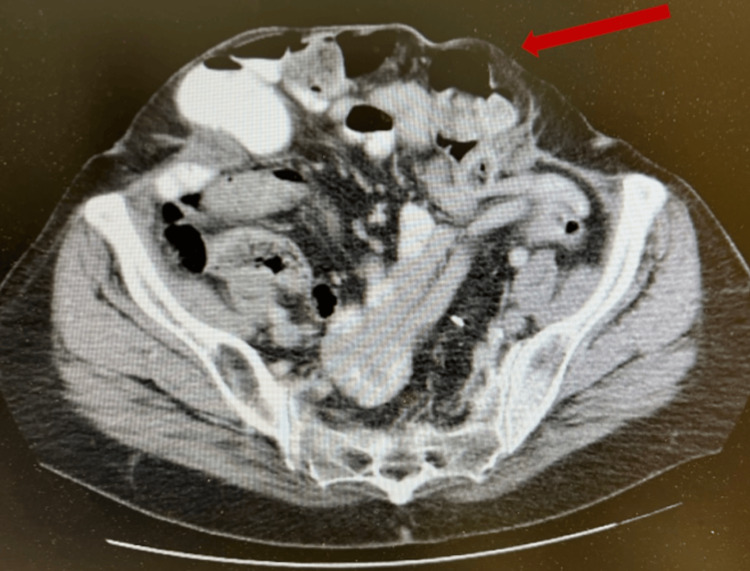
CT scan demonstrating a giant ventral hernia with significant loss of domain, characterized by protrusion of abdominal contents beyond the confines of the abdominal cavity Giant ventral hernia (red arrow) CT: computed tomography
